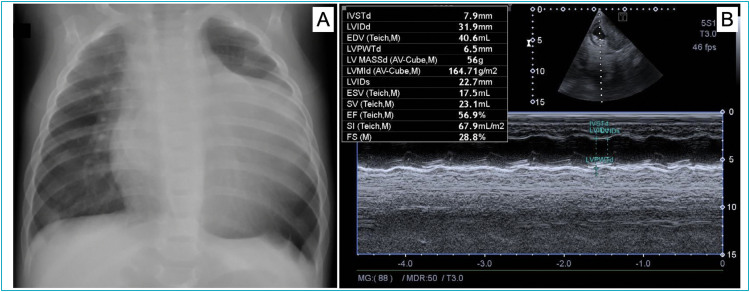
Chest radiography in posteroanterior view (A) demonstrating enlargement of the cardiac silhouette. Echocardiogram (B) showing a heterogeneous mass.
